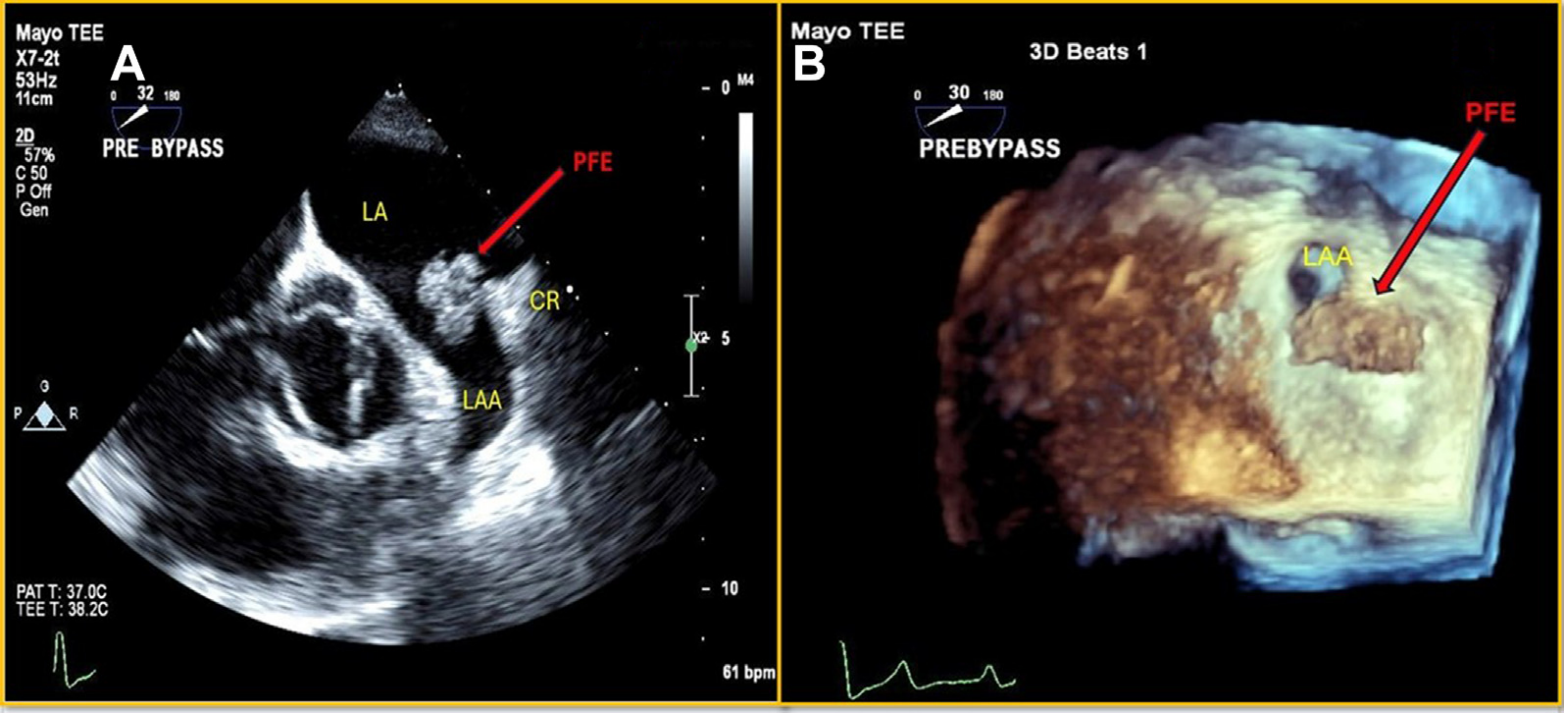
Transesophageal Echo (A) Intraoperative 2-D TEE, focus on LAA showing a mass attached to PVR. (B) Intraoperative 3-D TEE, focus on LAA showing a mass attached to PVR. Abbreviations as in Figures 1 and 2.
